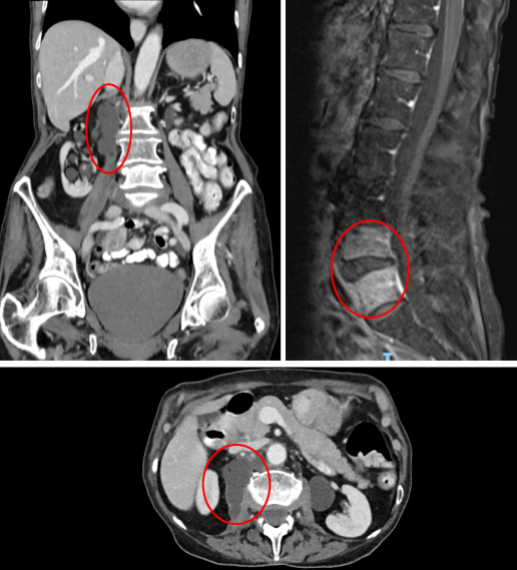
MRI scans showing psoas abscess and L4/5 discitis
